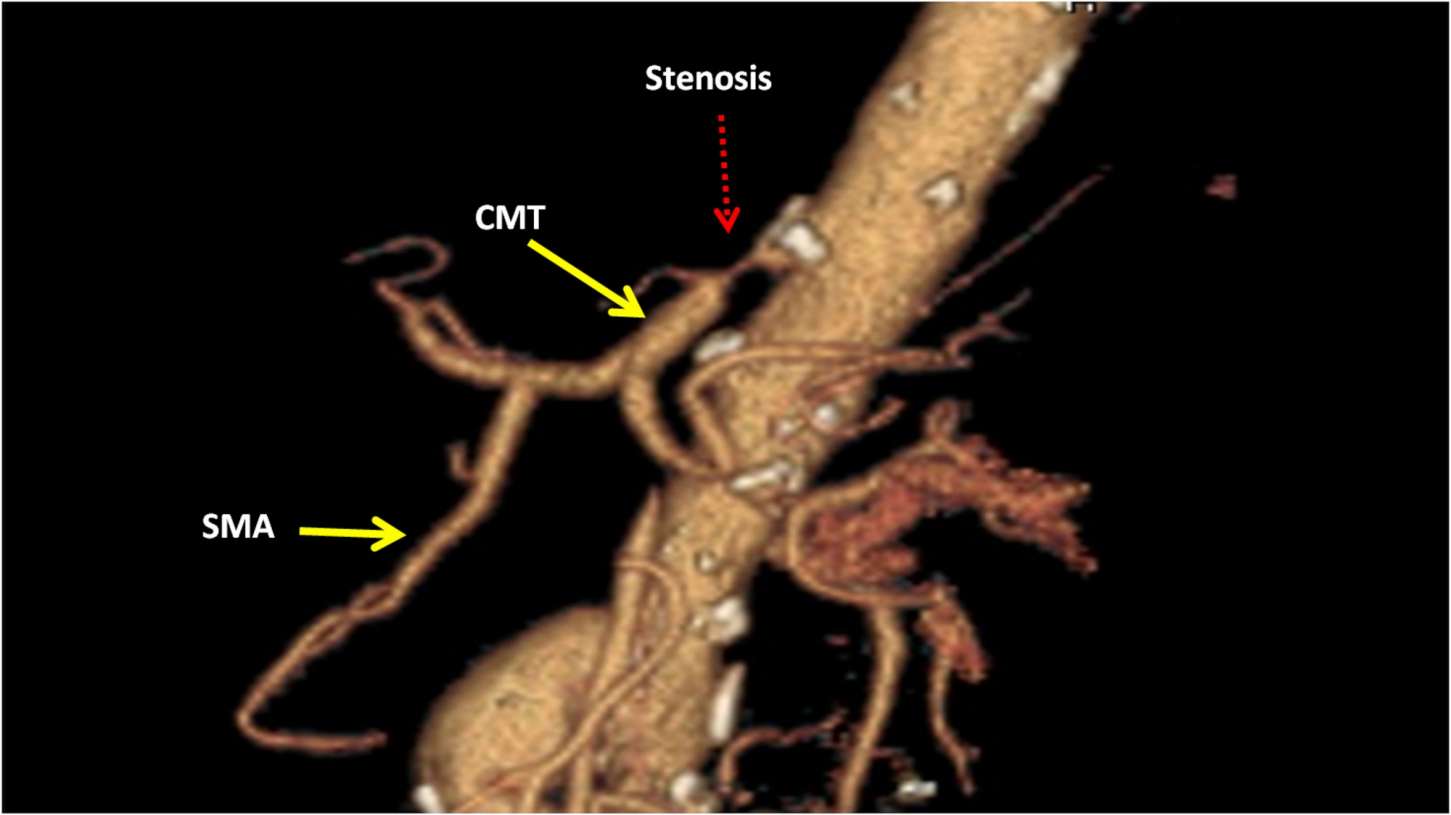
3D reconstruction of CT angiography of the abdomen showing rare CMT trunk with proximal stenosis. CMT: Celiomesenteric trunk; CT: Computed tomography; SMA: Superior mesenteric artery.
